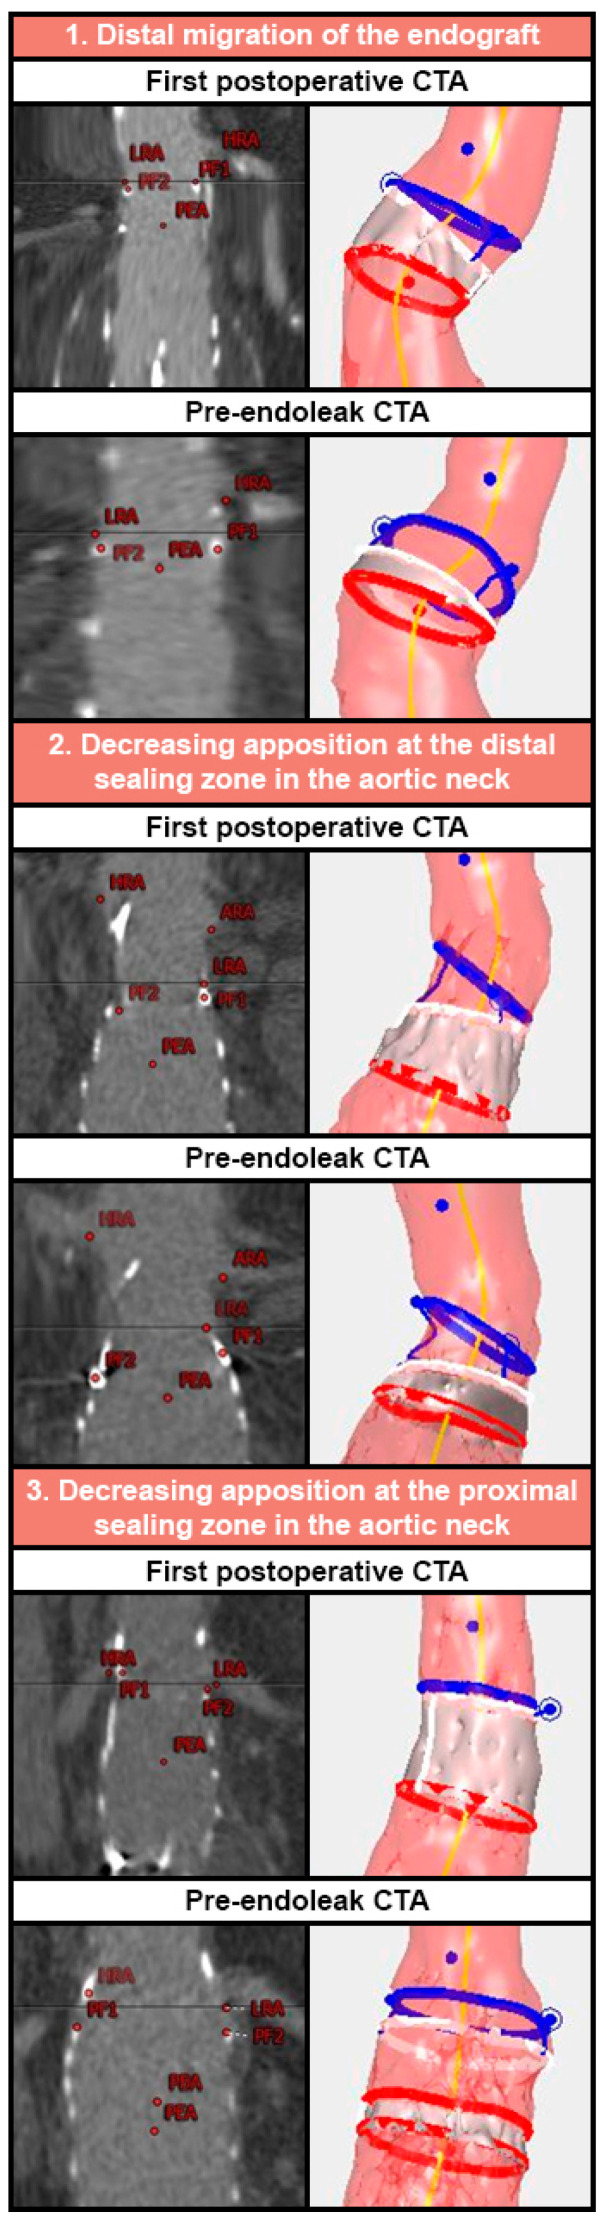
Examples of different mechanisms of decreasing sealing zone on computed tomography angiography (CTA) and the corresponding vascular imaging analyses (VIA) output. The blue circle represents the origin of the renal arteries, the white circle the endograft fabric, and the red circle the first slice where circumferential apposition is lost. Thus, the grey area represents apposition. ARA: accessory renal artery; HRA: highest renal artery; LRA: lowest renal artery; PBA: proximal beginning of apposition; PEA: proximal end of apposition; PF: proximal fabric marker.
